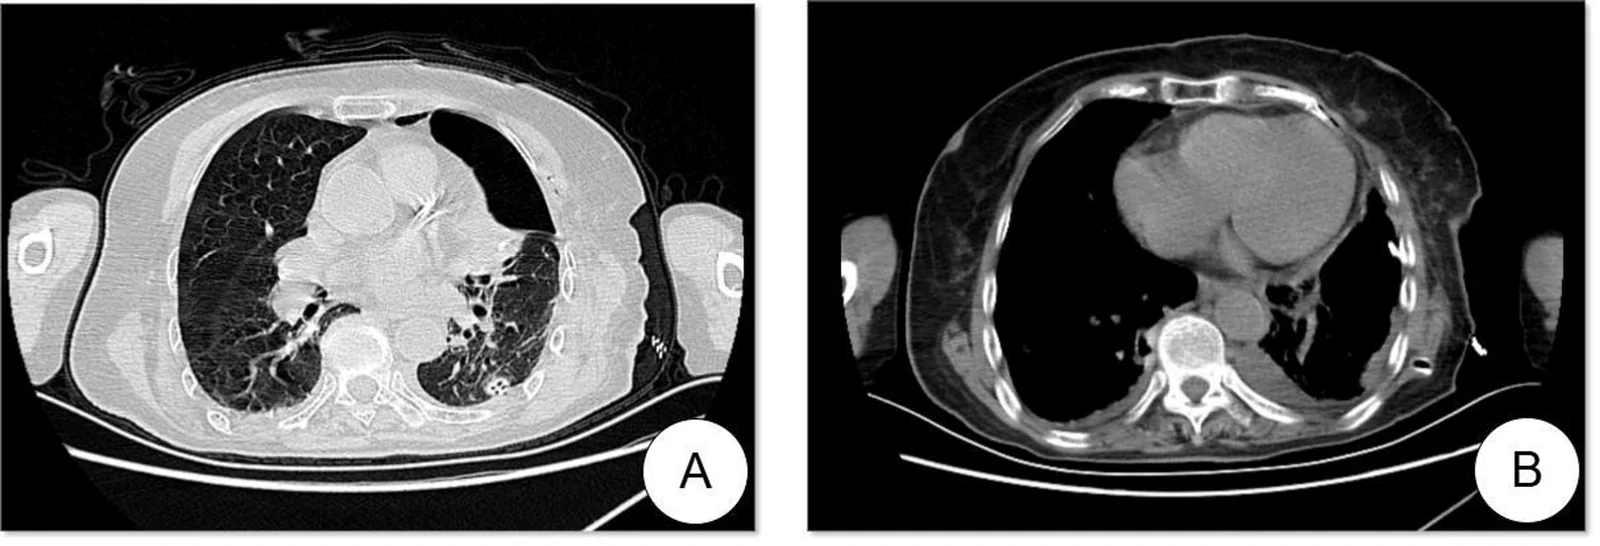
Noncontrast Chest Computed Tomography on Postoperative Day 2 After Left Upper-Lobe Sleeve Resection. (A) Lung window. (B) Mediastinal window. Left pleural air and fluid with a drainage catheter are present, consistent with postoperative change.
